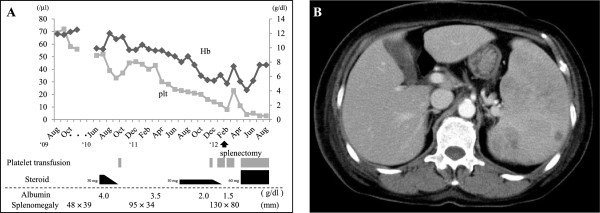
Summary of various clinical data before and after splenectomy, and the finding of an abdominal CT scan at surgery. (A) This patient showed prolonged idiopathic thrombocytopenia presenting with increased splenomegaly and non-response to steroid therapy. In addition, progressive pancytopenia, hypo-albuminemia, and hypo-γ-globulinemia coexisted. (B) The abdominal CT scanning revealed heterogeneously enhanced, severe splenomegaly without evidence of mass or nodular lesions.
